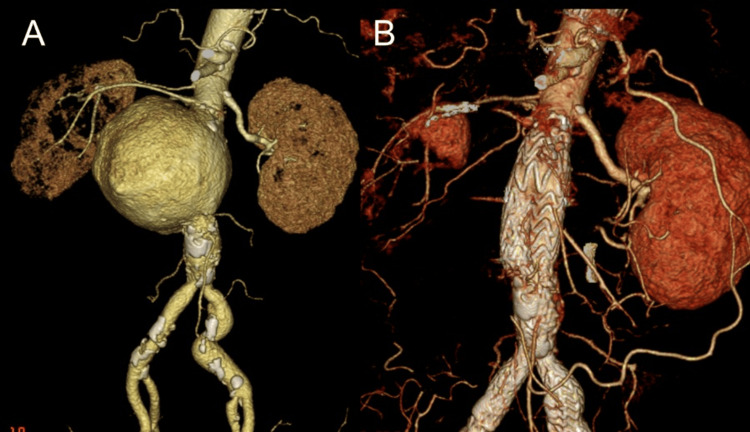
CT findings before and after emergency surgery (A) Preoperative contrast-enhanced CT showing a ruptured juxtarenal aneurysm and compression of the main trunk of the right renal artery. (B) Postoperative CT demonstrating successful aneurysm exclusion with no endoleak. The accessory renal artery is preserved, and right renal perfusion is maintained.
